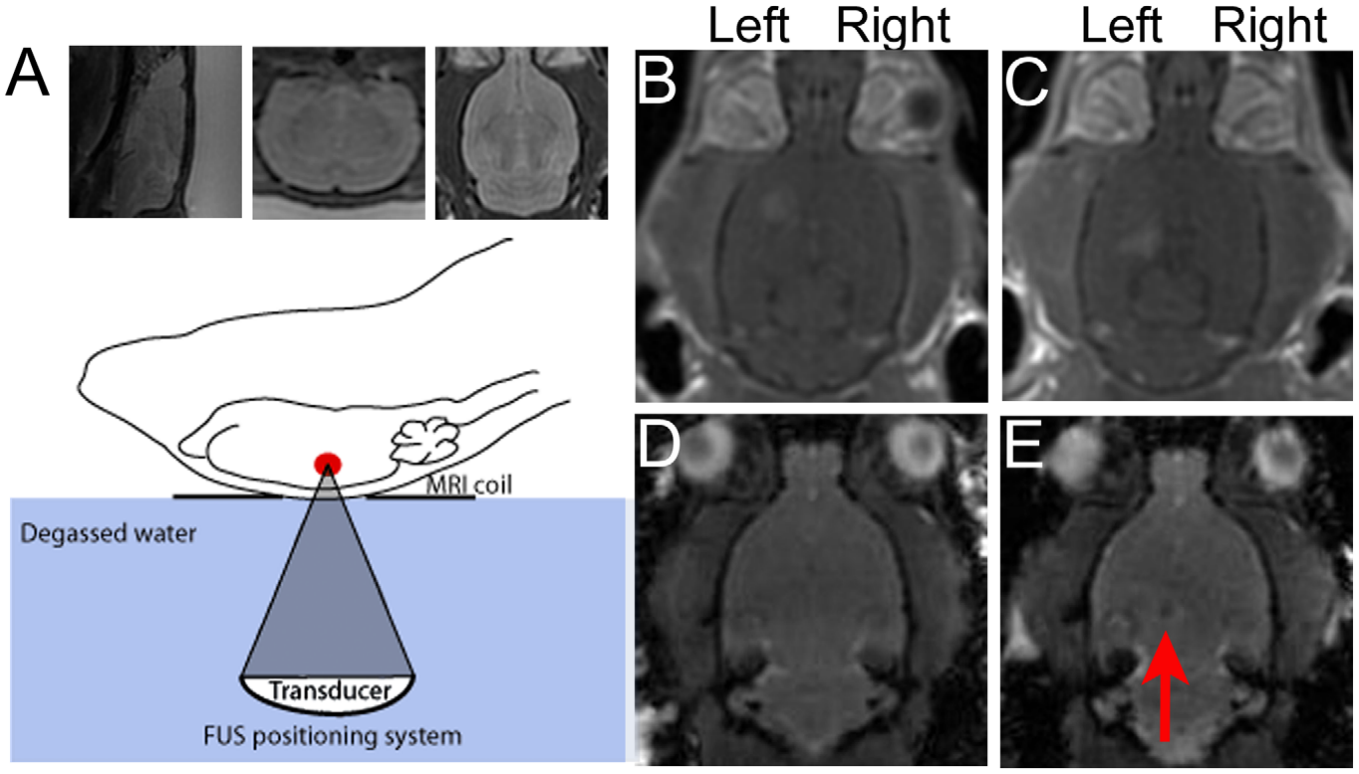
MRIgFUS increases BBB permeability to allow neural stem cells into the brain. A) T2-weighted MR images were taken in all 3 planes (sagittal, coronal and axial) and used to choose precise coordinates in the left striatum and left hippocampus for sonication with FUS. Contrast enhanced T1-weighted MR images were used to confirm increased BBB permeability in the striatum (B) and the hippocampus (C) prior to injection of neural stem cells into the carotid artery. Fast gradient echo (FGRE) sequences taken before (D) and 15 min after sonication (E) were used to confirm the entry of iron-labeled neural stem cells. Dark spots (red arrow) in the MR images taken after cell injection were used to confirm the presence of iron-labeled cells in the tissue.
